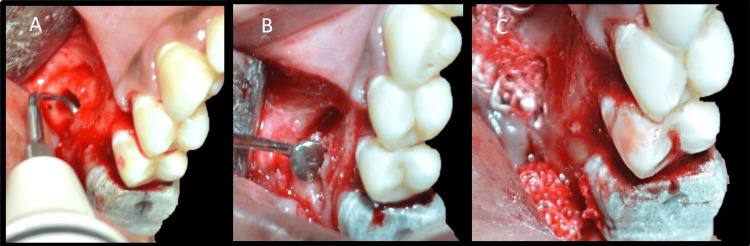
Retrograde preparation and filling A: Retro-preparation with a ultrasonic tip, B: Visualization through a microsurgical round mirror, C: Mineral trioxide aggregate condensation with a plugger
